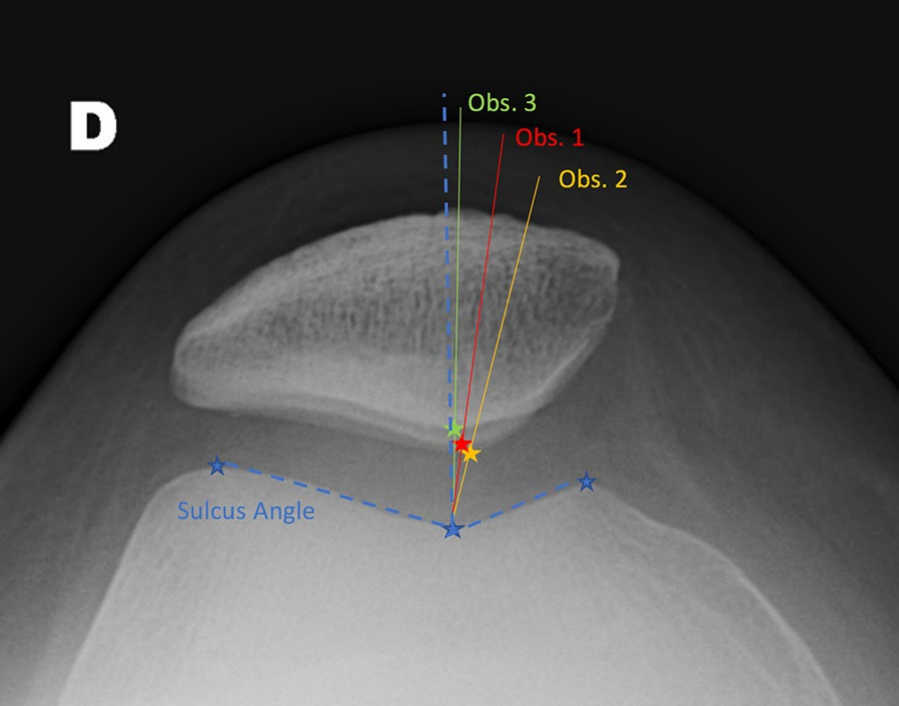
Radiograph of a Wiberg stage 3 patella case with intra- and interobserver variability in measurement
